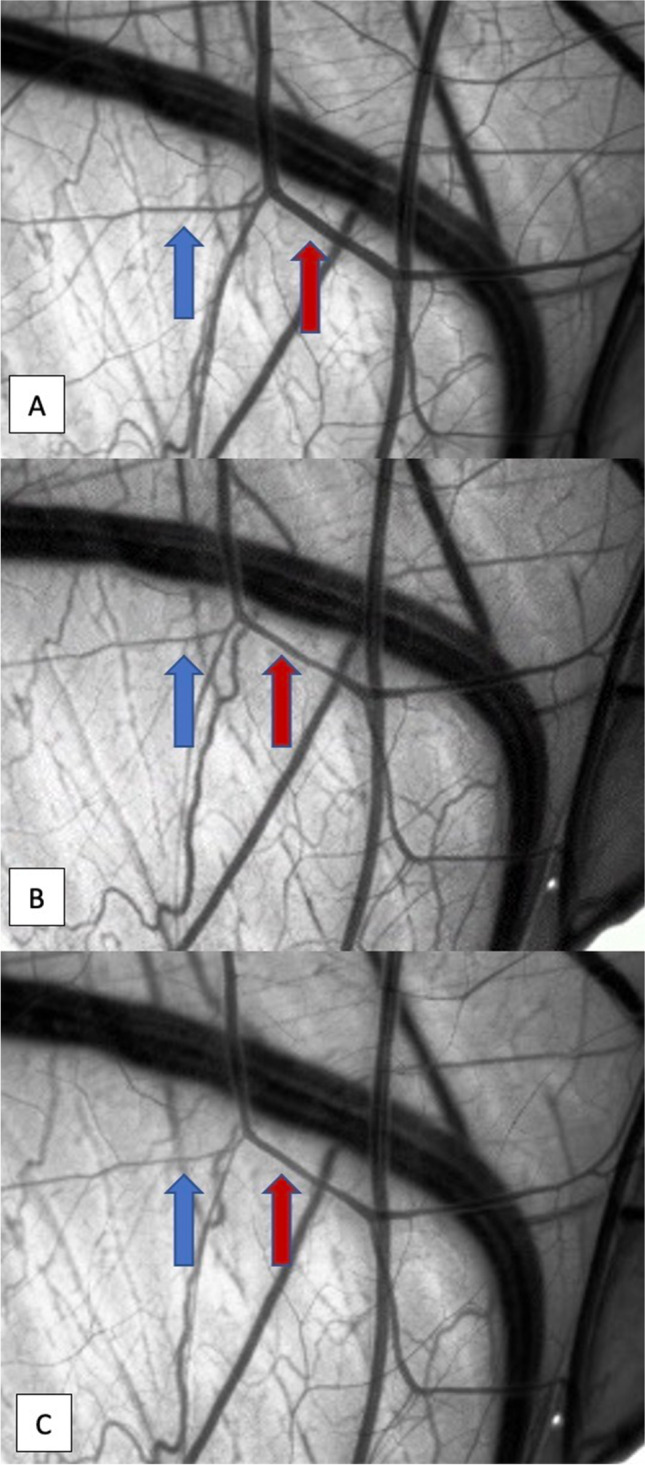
Visualization of the vasospasm development after ultrasound application for 60 s. (A) Vessel diameter before the ultrasound application; (B) first vessel diameter reduction 3 min after ultrasound application; (C) maximal vessel diameter reduction 8 min after ultrasound application
